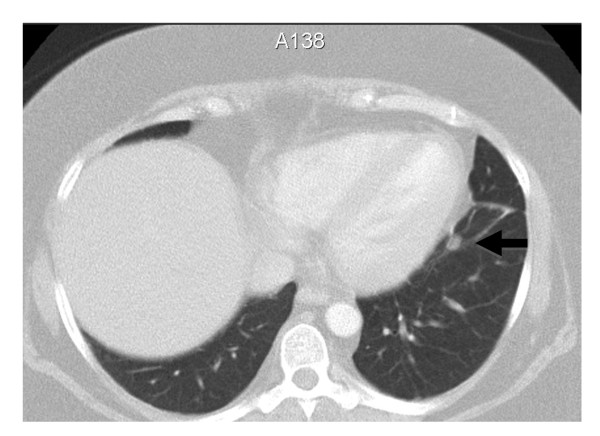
Transverse image of a chest CT scan showing a small 7 mm inferobasal segment lesion (arrow) in the left lower lobe.
